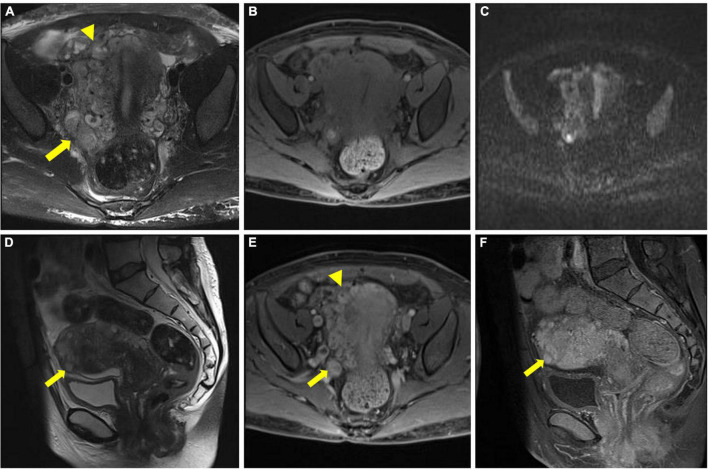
Pelvic magnetic resonance imaging (MRI) image. (A–C) Multiple tortuous and dilated vascular structures at the fundus of the uterus (arrowhead) and the parametrium (arrow). (D) Multiple nodules of varying sizes were observed at the fundus of the uterus (arrow). (E,F) After gadolinium-based contrast material injection, progressively enhanced lesions were noted within the lumen (arrowhead and arrow in E), and the nodules also presented progressive enhancement (arrow in F).
